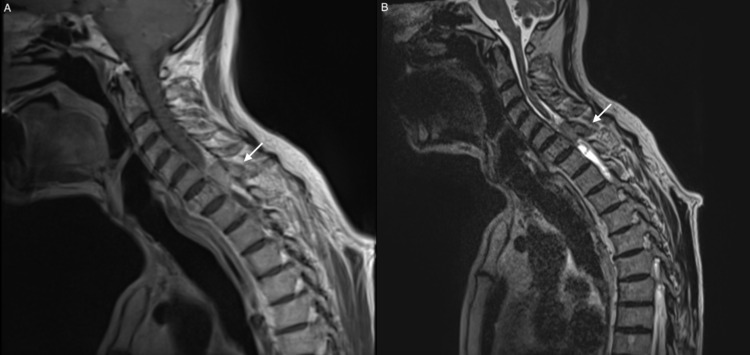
Sagittal MRI of the spine. (A) T1-weighted sagittal MRI shows injury of the vertebral body of C7 and presence of a non-enhancing extramedullary tumor measuring 4.19 cm. (B) T2-weighted sagittal MRI shows a hypointense extramedullary tumor MRI, magnetic resonance imaging
